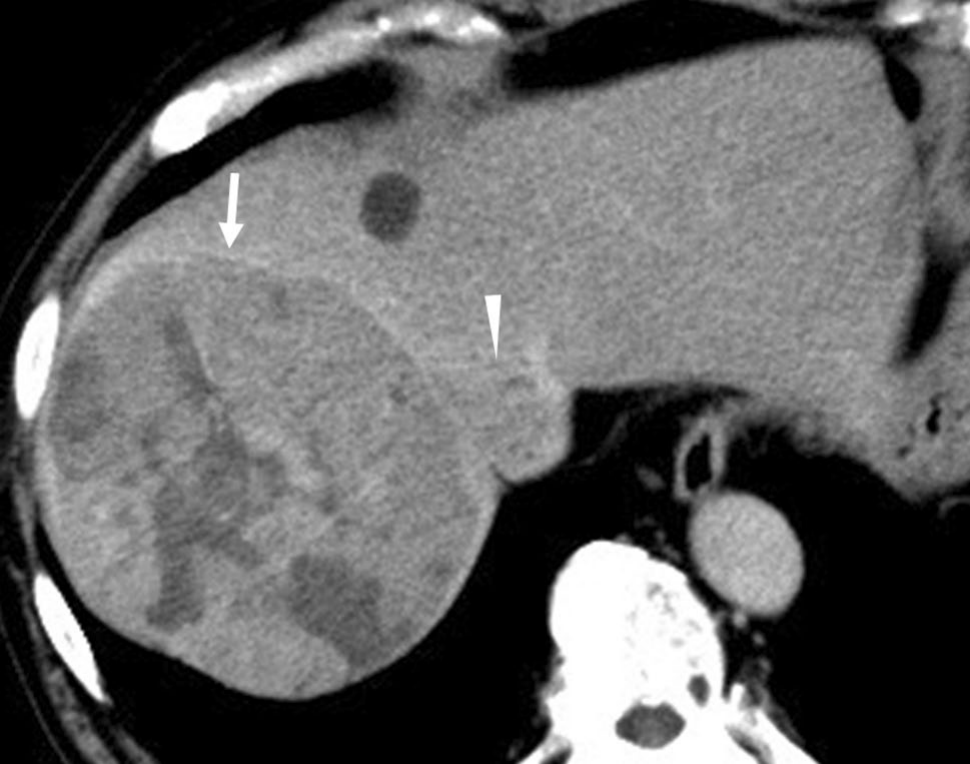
CT findings at initial diagnosis. Shown are the CT images from the patient’s first visit to his previous hospital. A solitary tumor, 13 × 9.5 cm in diameter, can be seen in segments 8 and 7 (arrow). IVC-HVTT, 18 × 20 mm in diameter (arrowhead), can be clearly seen from the right hepatic vein. *CT* computed tomography, *IVC-HVTT* hepatic vein tumor thrombosis protruding into the inferior vena cava
